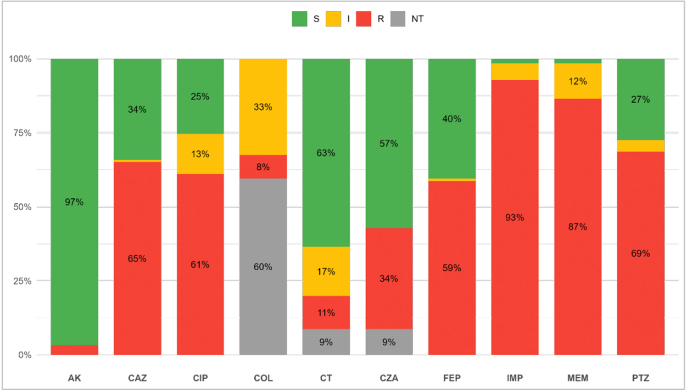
Antibiotic susceptibility results . Antimicrobial susceptibility testing performed for the 126 isolates by automated broth microdilution using the Vitek 2.0 Compact system (bioMérieux). CAZ: Ceftazidime; FEP: Cefepime; IPM: Imipenem; MEM: Meropenem; PTZ: Piperacillin/tazobactam; AK: Amikacin; CIP: Ciprofloxacin; COL: Colistin; CZA: Ceftazidime/avibactam; CT: Ceftolozane/tazobactam. S: Susceptible; R: Resistant; I: Intermediate. Interpretation according to CLSI 2024 breakpoints. N/T: Not tested.
